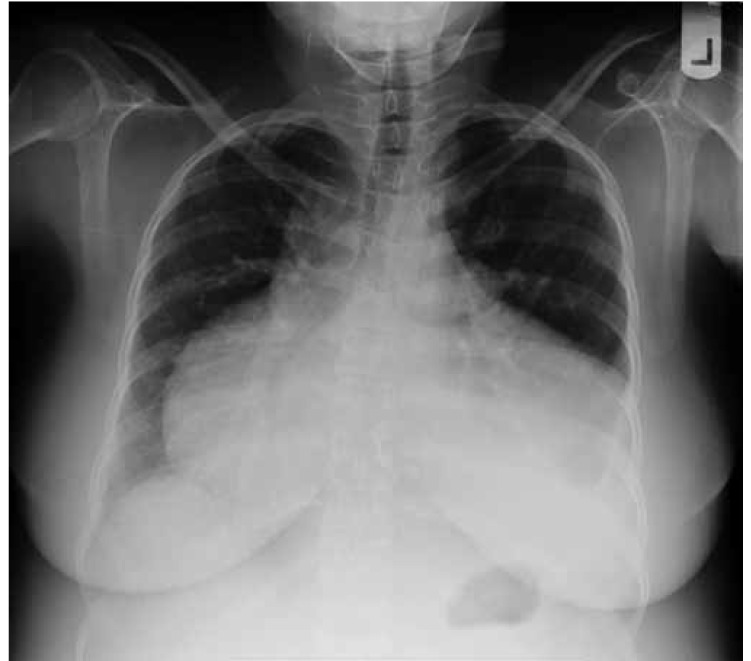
Chest X-ray postero-anterior view.
